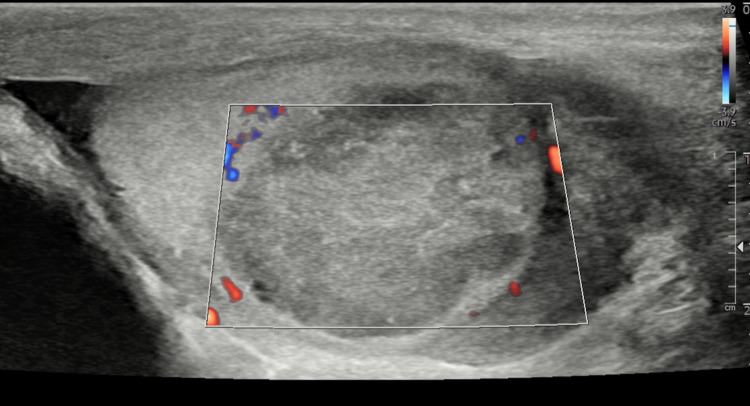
Ultrasound testes of the avascular left testicular lesion demonstrated by absent colour Doppler flow
